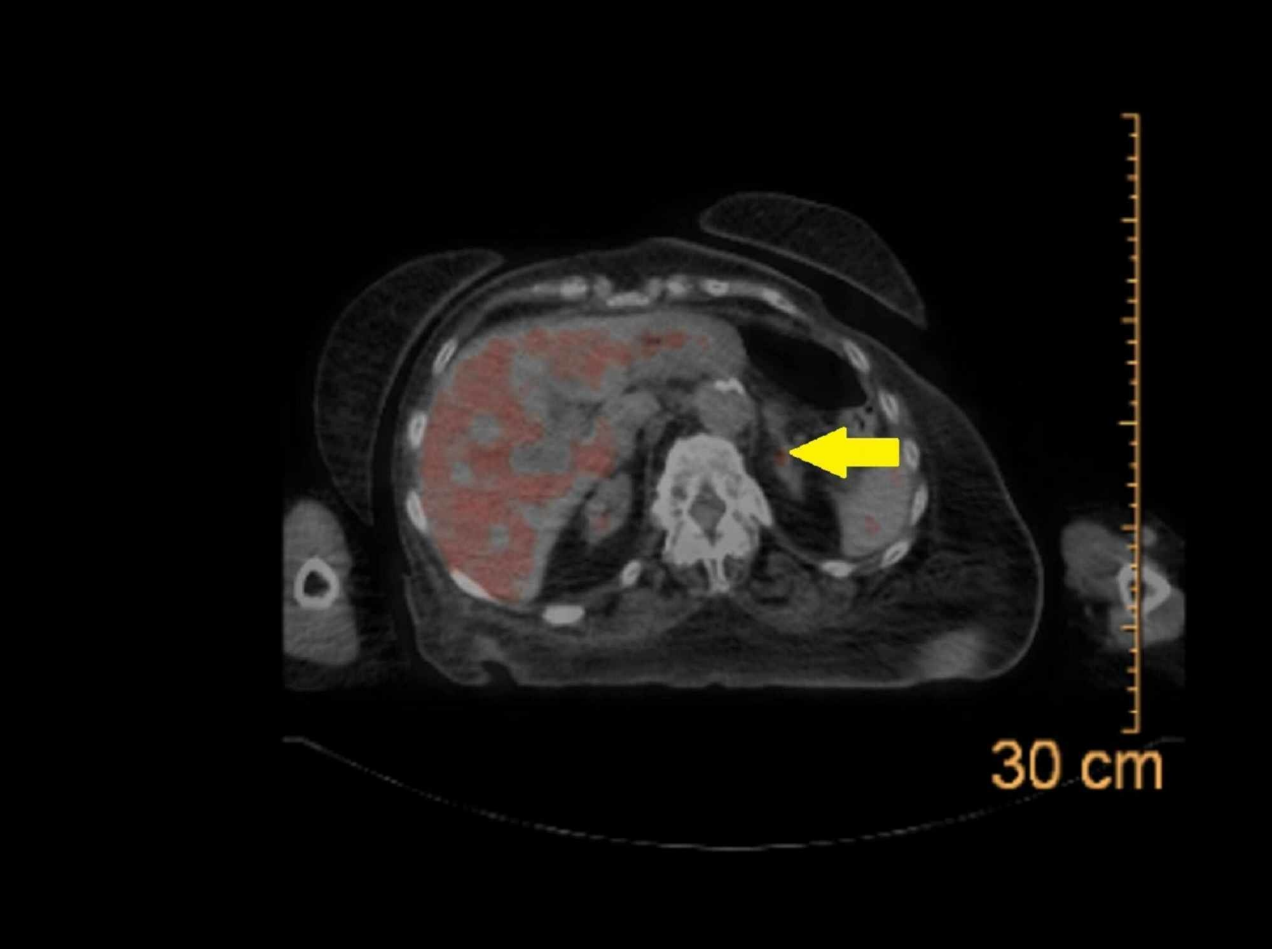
After administering FDG infusion, PET-CT scan demonstrated a metabolically active prominent left adrenal gland with a maximum SUV of 4 and a volume of 1.6 cc. FDG, 18F-fluorodeoxyglucose; PET, positron emission tomography; SUV, standardized uptake value.
